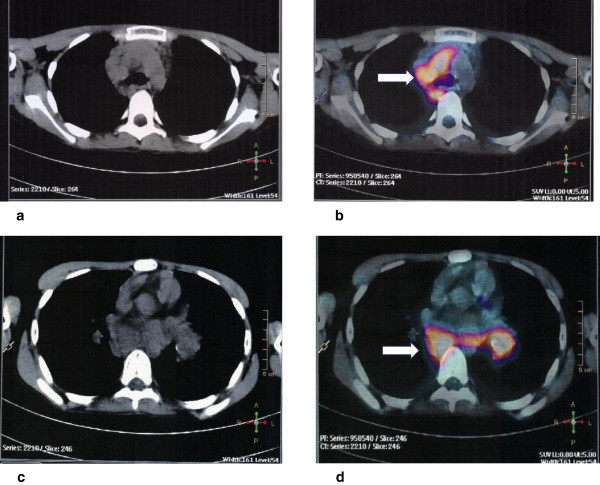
(a-c) Axial contrast-enhanced computed tomography image of the mediastinal region, and (b-d) fused transverse ^
